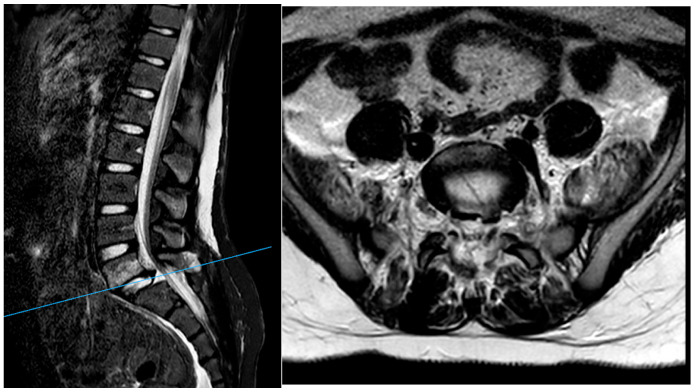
MRI showing the rupture of the L5–S1 disc with disruption of the anterior and posterior longitudinal ligaments and the interspinous and supraspinous ligaments.
